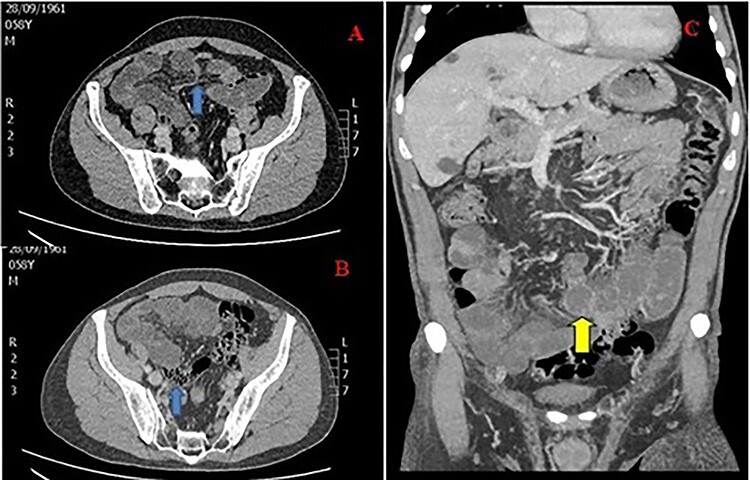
CT of the abdomen in a 59-year-old male with SBO; (A) transition point in the right iliac fossa (arrow), (B) feces sign (arrow) and (C) close loop obstruction (arrow).
